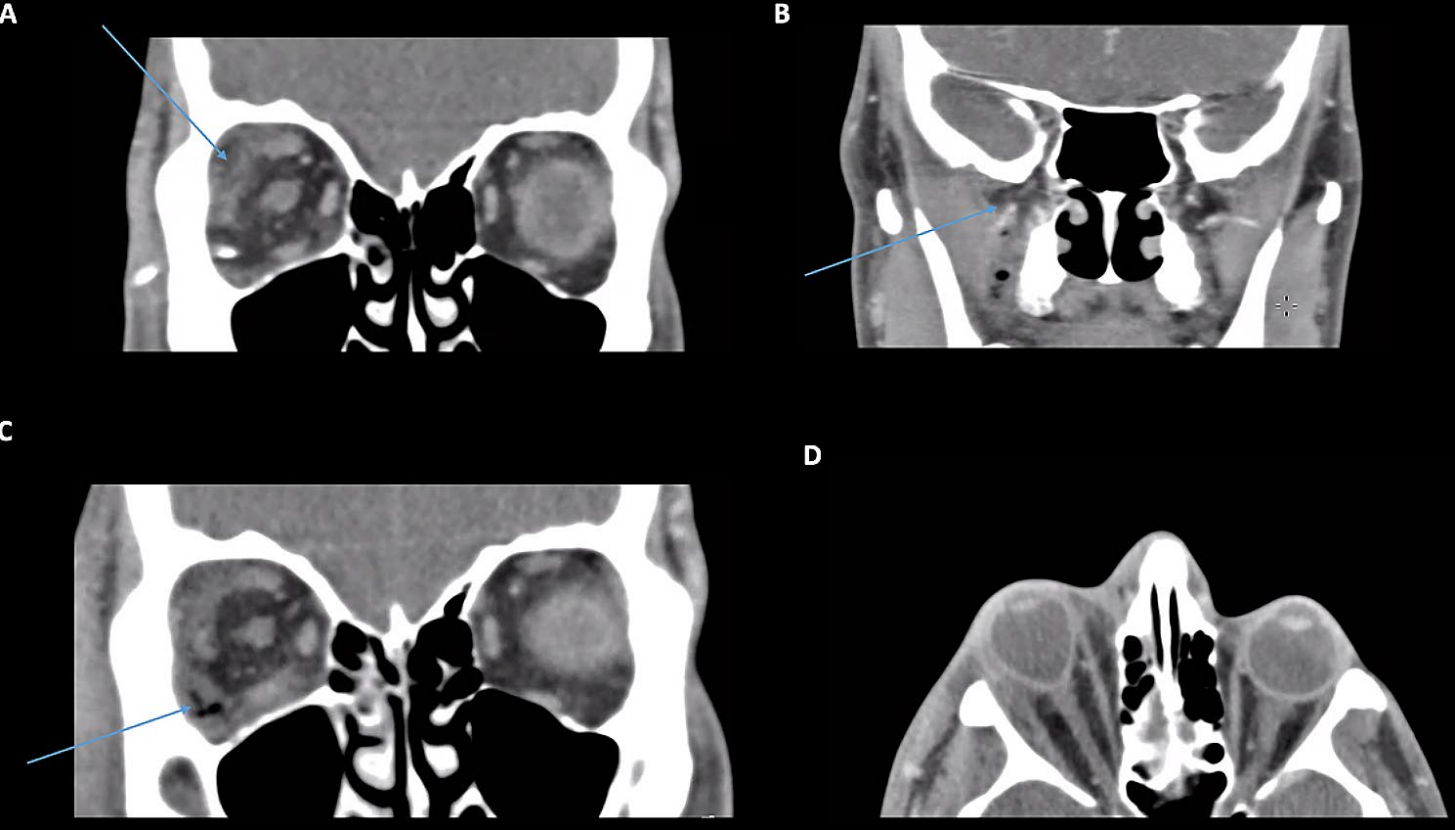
Case 1: CT orbital scans. A: Coronal image highlighting extensive subperiosteal abscess involving the right superolateral and inferolateral regions of the orbit. B: Soft tissue enhancement with alteration of the fat signal within the pterygopalatine and infratemporal fossae. C-D: A repeat CT orbital scan 2 days later demonstrating the presence of orbital emphysema with proptosis and tenting of the right globe
